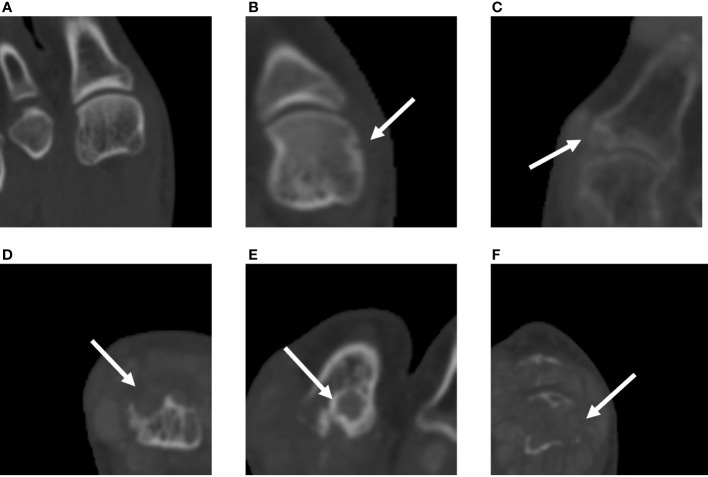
Assessment of bone erosion in the MTP joints with CT images using the modified SvdH erosion scoring method. (A) A score of 1 indicates tiny and discrete erosion. (B) A score of 2 indicates spread erosion. (C) A score of 3 indicates an erosion surface nearly half of the joint surface. (D) A score of 4 indicates erosion exceeding half of the joint surface. (E) A score of 5 indicates extensive bone erosion and loss. SvdH, Sharp/van der Heijde; CT, computed tomography; MTP, metatarsophalangeal joint.
